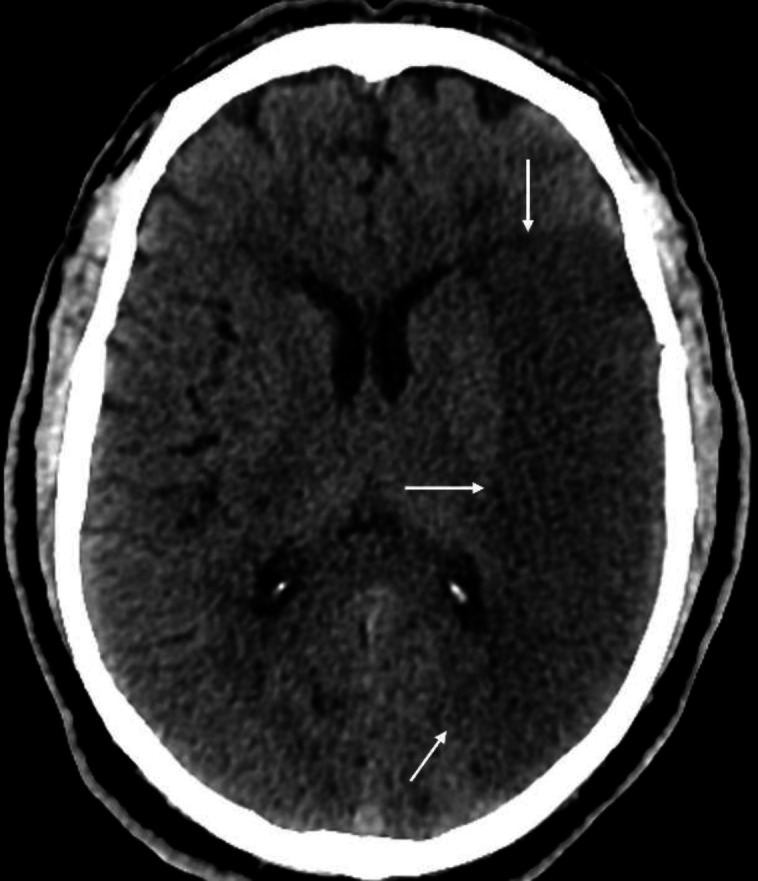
Non-contrast axial CT brain of a 55 years-old female with acute ischemic infarction involving the left middle cerebral artery territory. Patient presented with right hemiplegia that evolved over past 9 hours. Large ill-defined low density area (arrows) involving the left parietal lobe and causing effacement of the adjacent sulci is the characteristic finding of an acute ischemic infarction on non-contrast CT. Note absence of any significant mass effect
